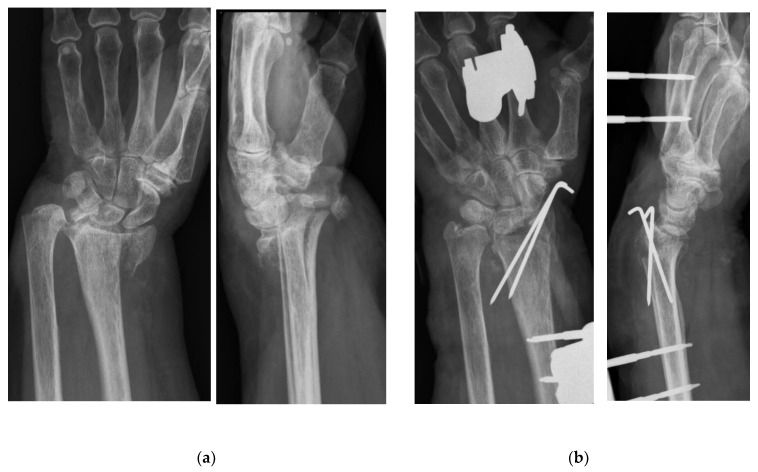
X-rays in posterior-anterior (left) and lateral (right) view after the initial patient’s presentation (a) as well as after K-wire and an external fixation installation (b).
